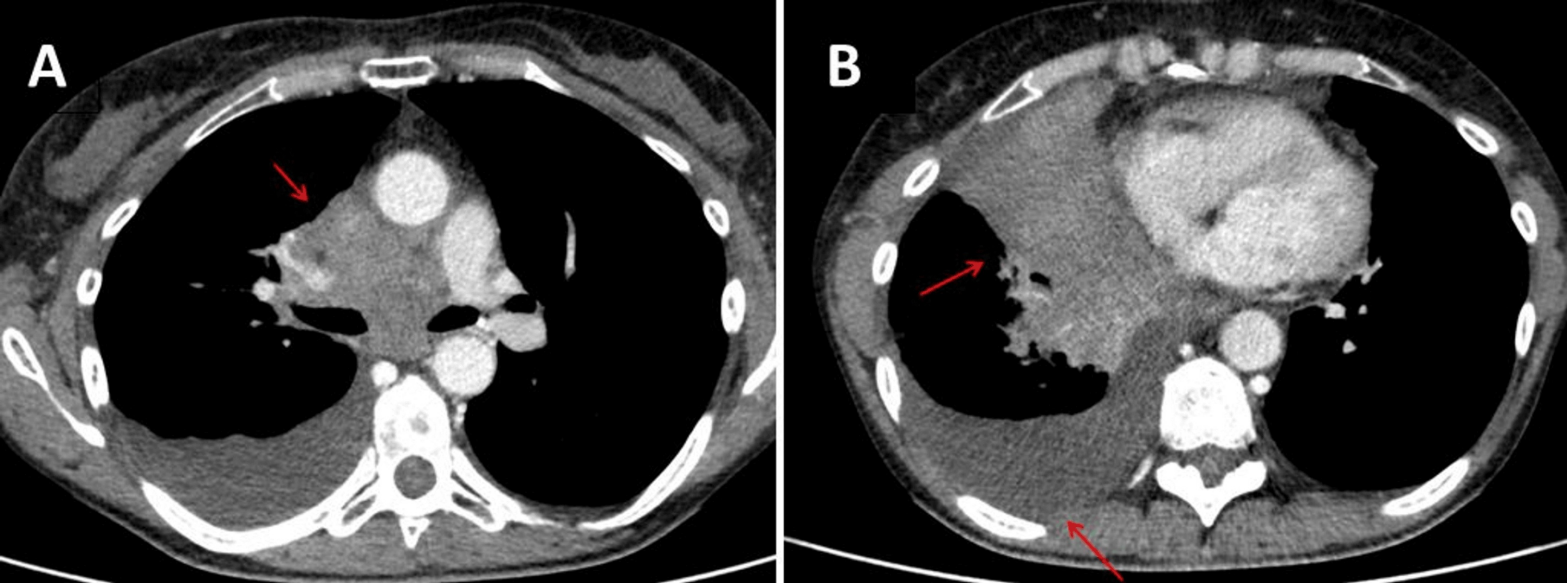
CT scan of the chest showing a central mass compressing the superior vena cava (A). Tumor growth was also complicated by pleural effusion and atelectasis of the middle lobe bronchus (B).
